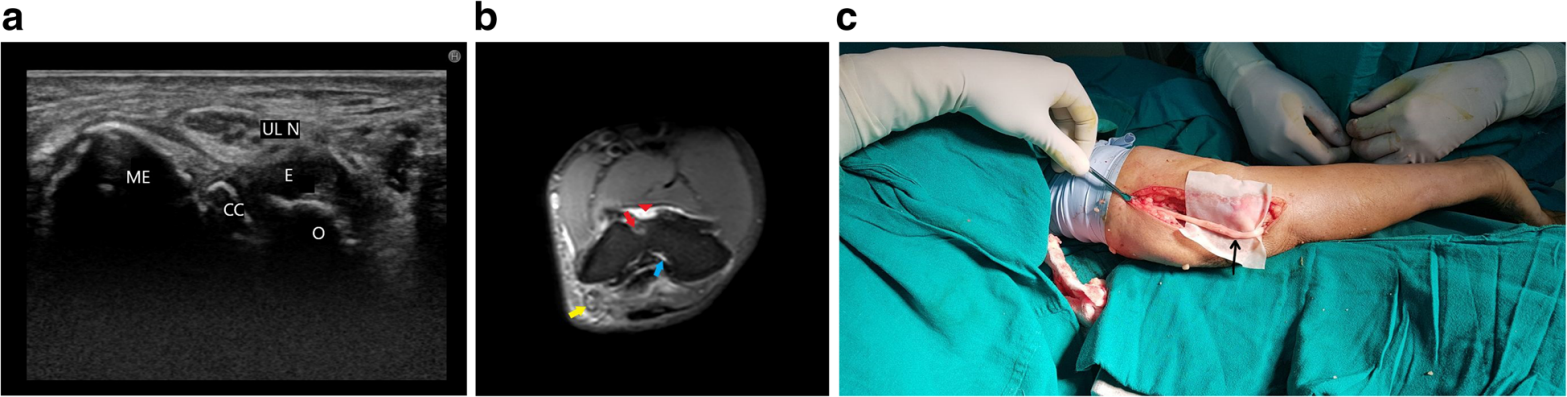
a Grey scale ultrasound image shows a hypoechoic, significantly thickened ulnar nerve (ULN) in the cubital tunnel with underlying joint effusion (E) and cortical irregularity (CC). The medial epicondyle (ME) and olecranon process (O) forming boundaries of the cubital tunnel are also seen. b T2-weighted MR image of the patient shows hyperintensity of the ulnar nerve (yellow arrow) with surrounding arthritic changes—subchondral cyst (red arrow), marrow oedema with cortical irregularity (blue arrow) and joint effusion (red arrowheads). c Intra operative picture of the patient showing the thickened ulnar nerve (black arrow)
